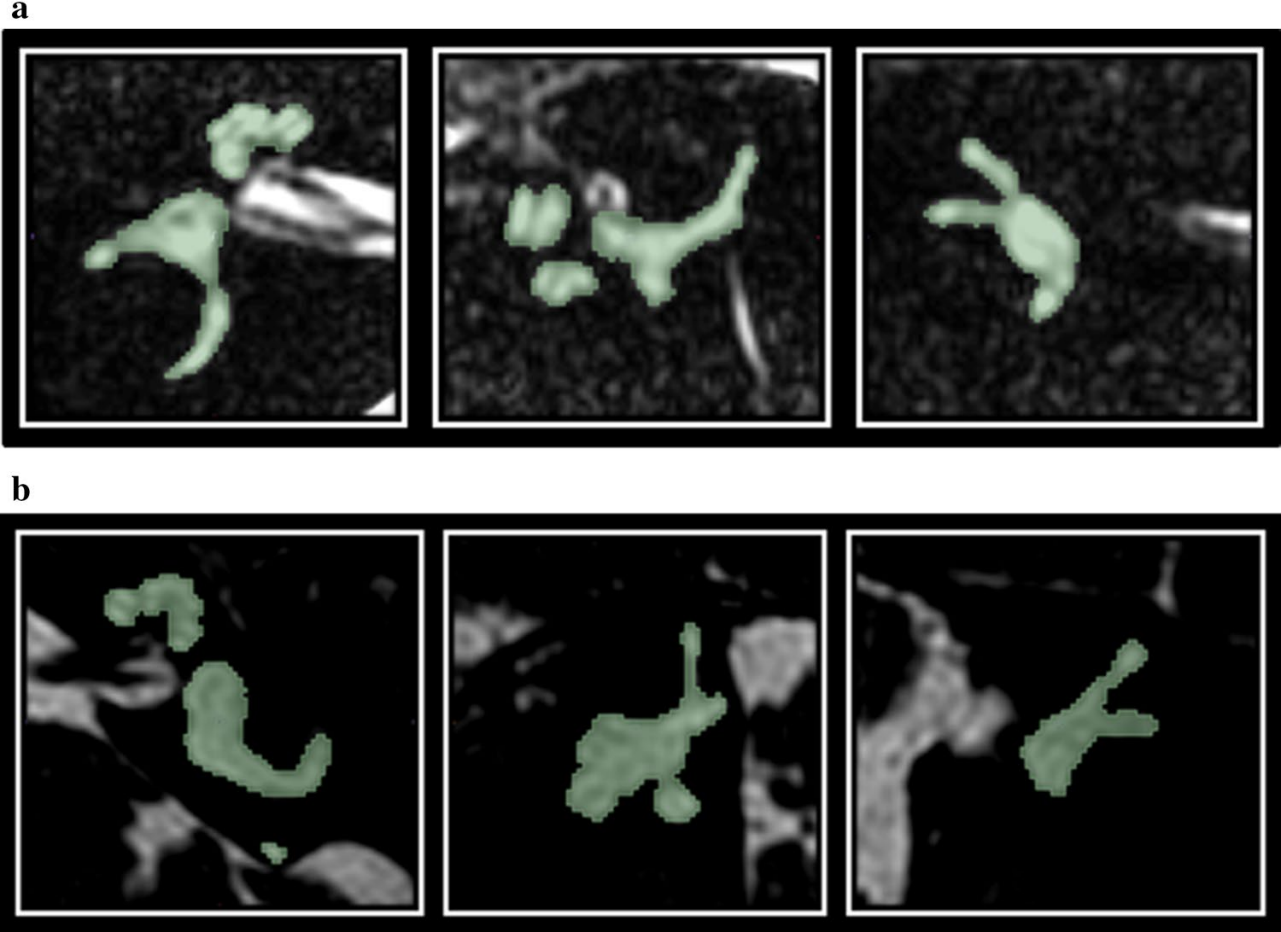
a A cropped MR image of a right inner ear of a subject with asymmetric sensorineural hearing loss on the right side. From left to right, the axial, sagittal and coronal planes are presented. The manual segmentation is visualized by the green mask b A cropped MR image of a left inner ear of a subject with unilateral Menière’s disease on the left side. From left to right, the axial, sagittal and coronal planes are presented. The manual segmentation is visualized by the green mask
